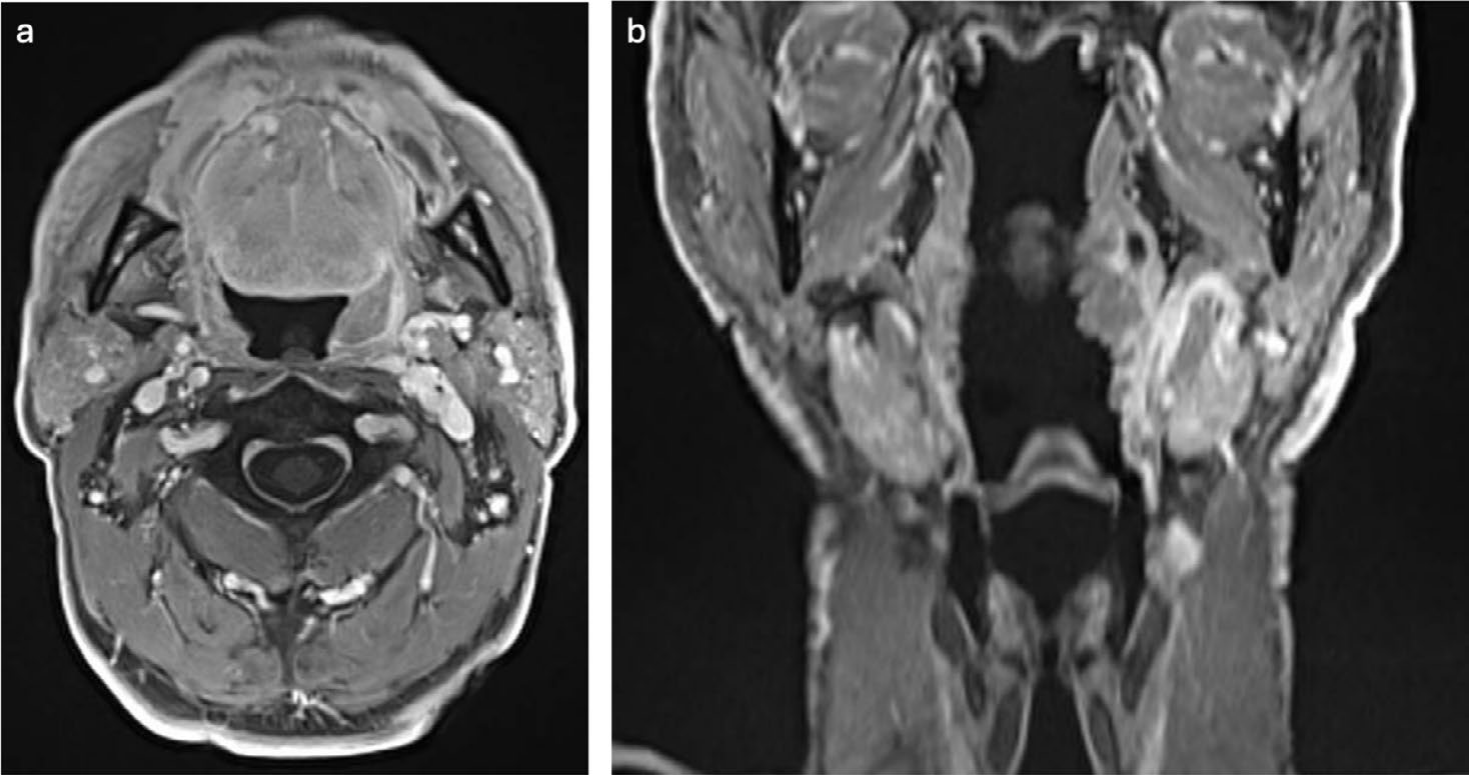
Preoperative MRI scan. (a: axial section; b: coronal section).
